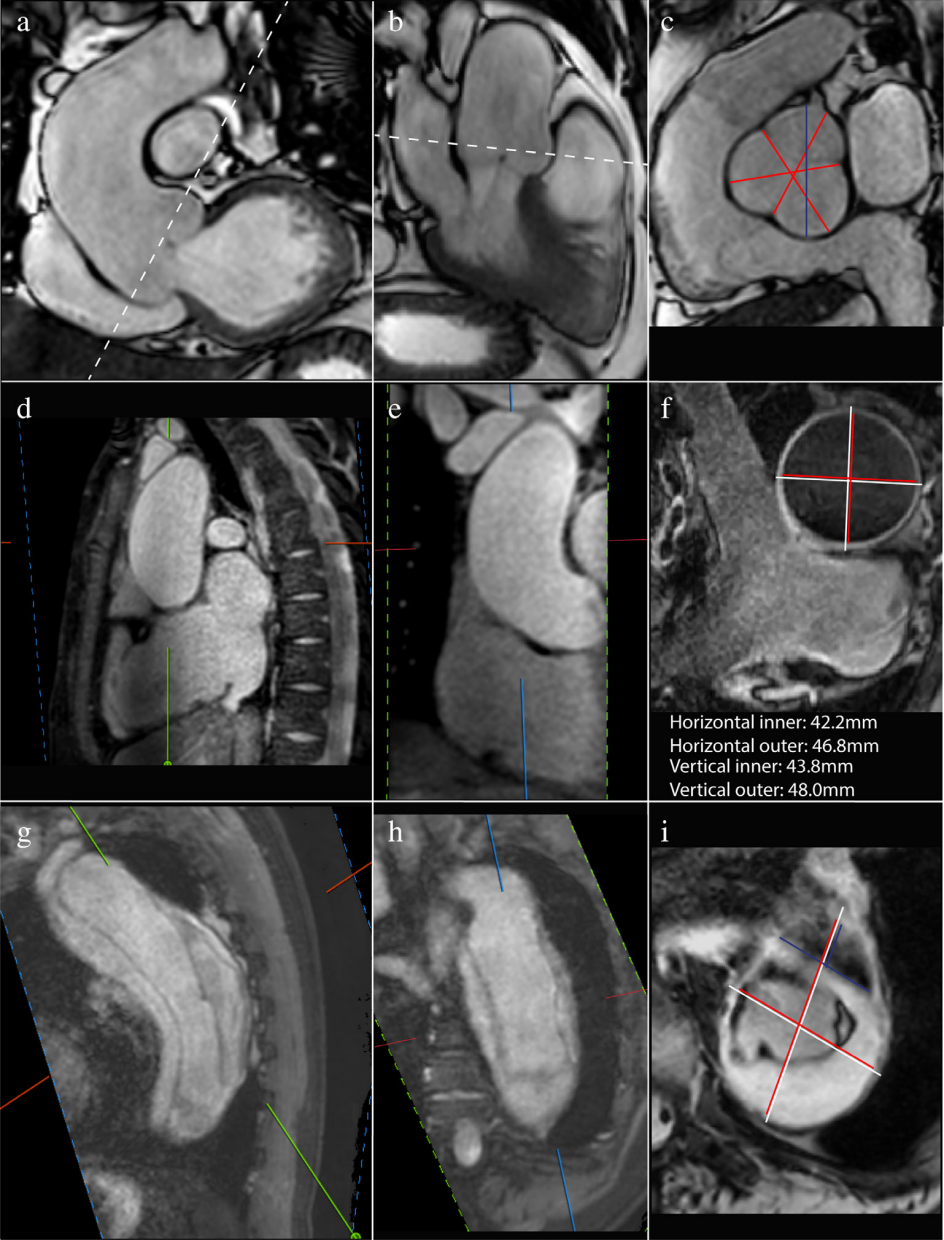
MRI images of pathologic aorta's. Top three images: dilated sinus planned on (a) coronal and (b) sagittal views for (c) double‐oblique I‐I measurement (SSFP cine only provides luminal enhancement, vessel wall not clearly deliniated): average cusp‐to‐commissure (in red) and largest cusp‐to‐cusp diameter (in blue). Three middle images: bicuspid valve with dilated ascending aorta with MRA planning views (d) and (e,f) Black‐blood double‐oblique measurement (red: I‐I, white: O‐O). Three images below: type B aortic dissection with mural thrombus, MRA planning views (g–i) Black‐blood double‐oblique measurement (red: I‐I, white: O‐O, blue: true lumen, crossing of red and white lines: false lumen surrounded by mural thrombus).
